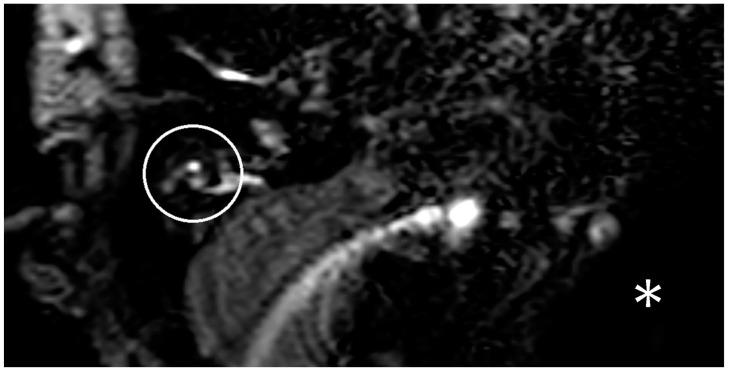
A depiction of a three-dimensional fluid-attenuated inversion recovery (3D-FLAIR) sequence with delayed contrast of the MRI of patient three. The right inner ear is marked with a white circle. There is also an artifact on the implanted left side, which is denoted with a white star. There is no hydrops on the not-implanted side.
